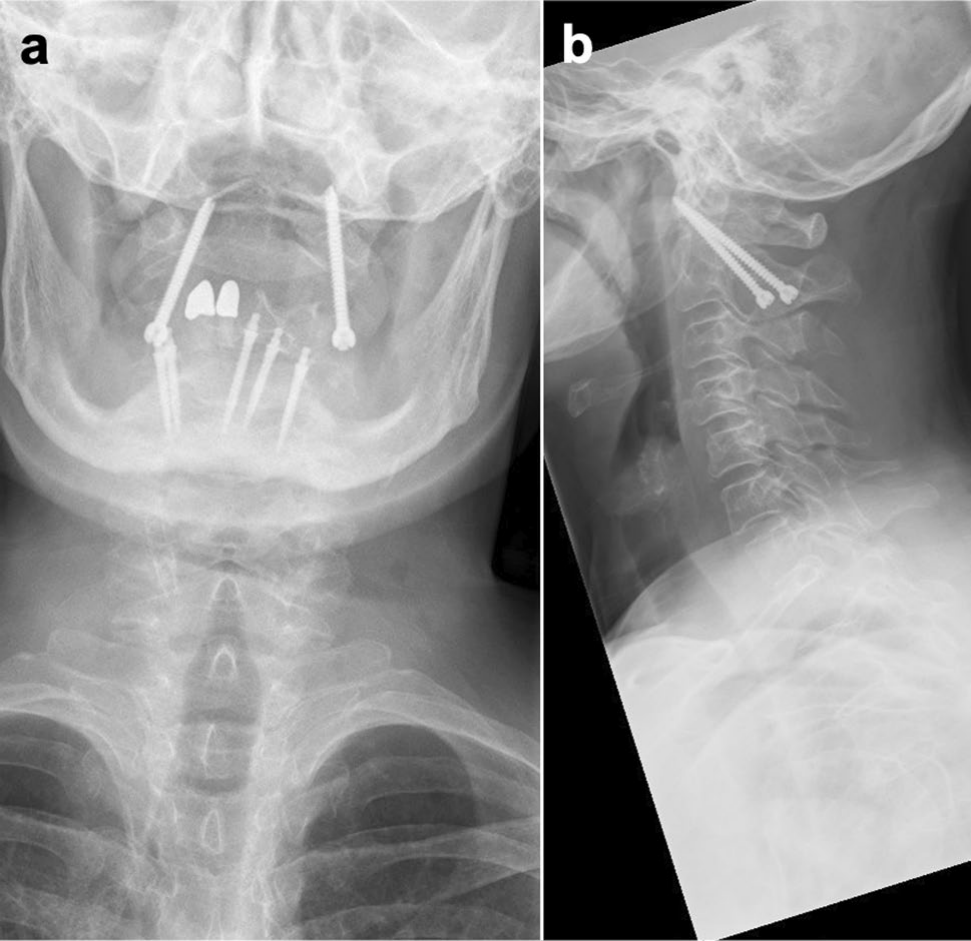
Incorrect screw position. This figure shows the malpositioning of a screw that occurred in the current study. Conventional X-ray. (a) Anterioposterior plane. The right-sided screw misses the articular surface of C1 on the medial side. No bridging of the joint occurs and the screw does not find a position in the right lateral mass of C1. (b) Sagittal plane. In this plane, the screw position appears largely correct.
